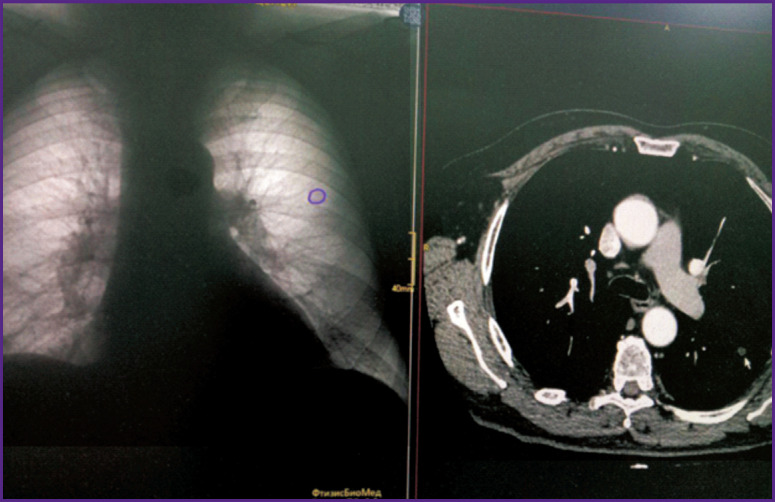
Prophylactic fluorography processing using PhthisisBioMed service (*left*) and CT scan results (*right*)
